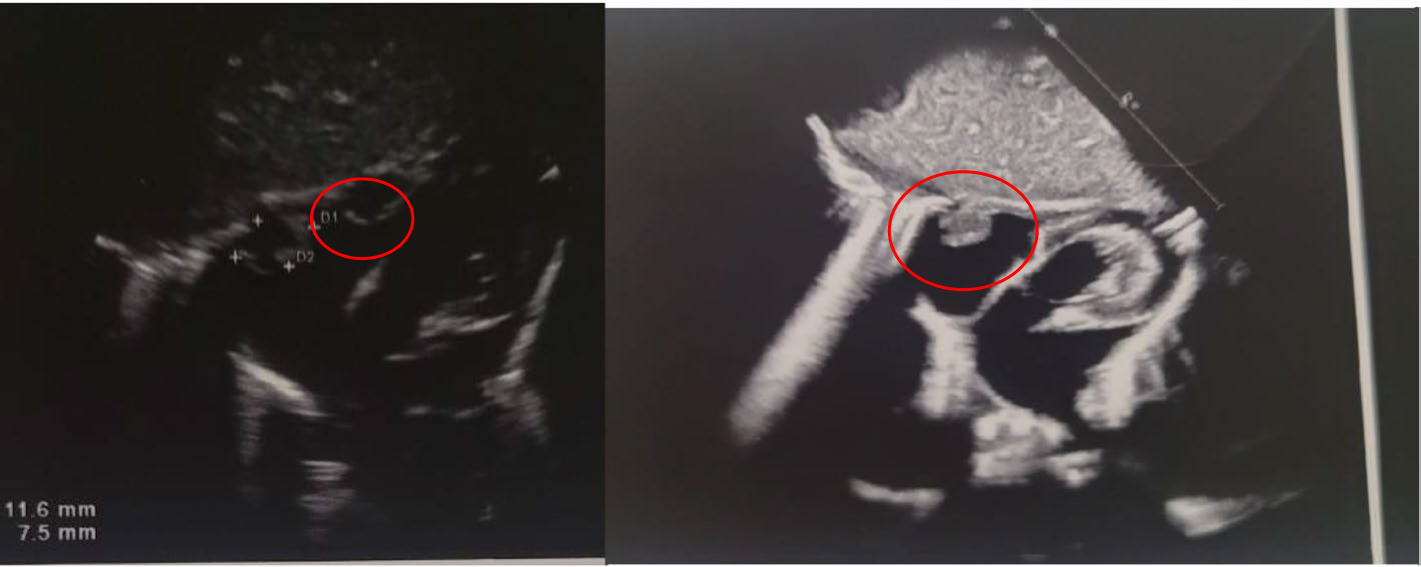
Echocardiogram: A hyperechoic formation with regular edges (1.5 × 1.5 cm) was found in the right atrium
